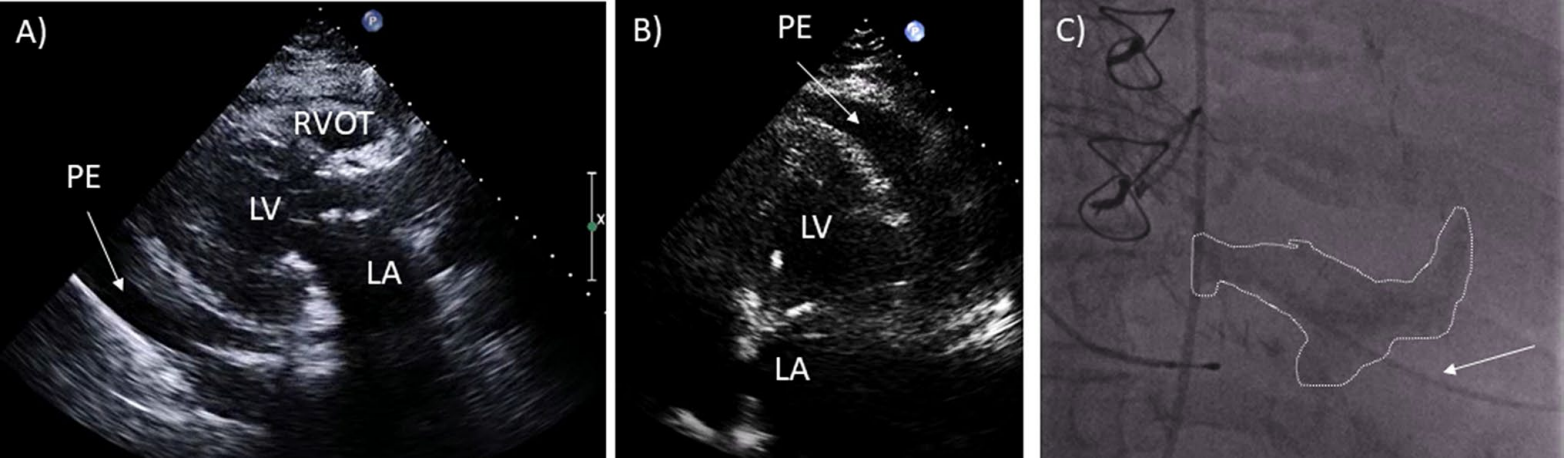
Pericardiocentesis. Posterior and lateral localized pericardial effusion (a, b). Echocardiography-guided apical puncture confirmed by contrast dye (dotted area) via a 5F dilatator (c, arrow). *LA* left atrium, *LV* left ventricle, *RVOT* right ventricular outflow tract, *PE* pericardial effusion
